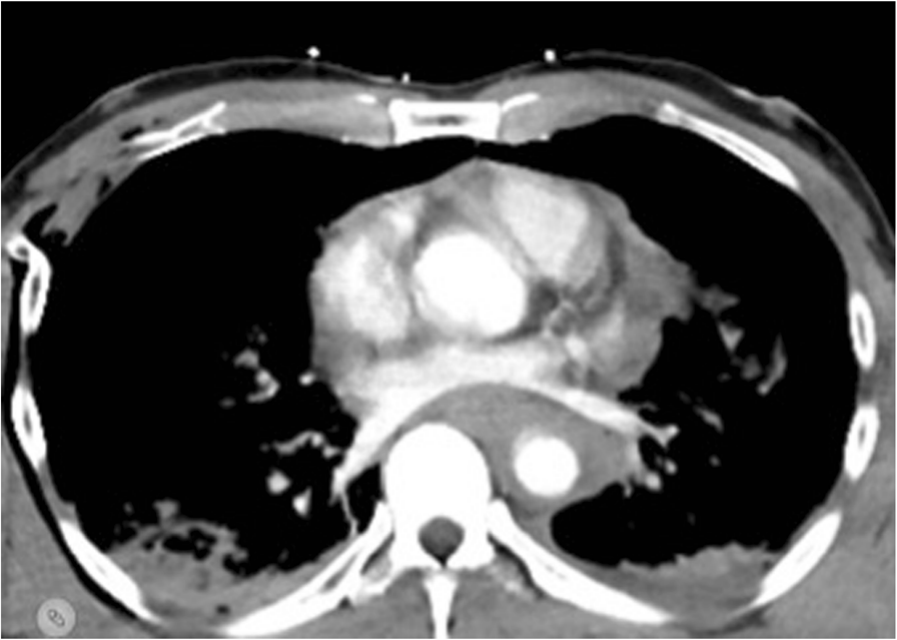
CT Thorax after contrast dye injection showing the rupture of ascending aorta. Arrow highlights aortic wall
